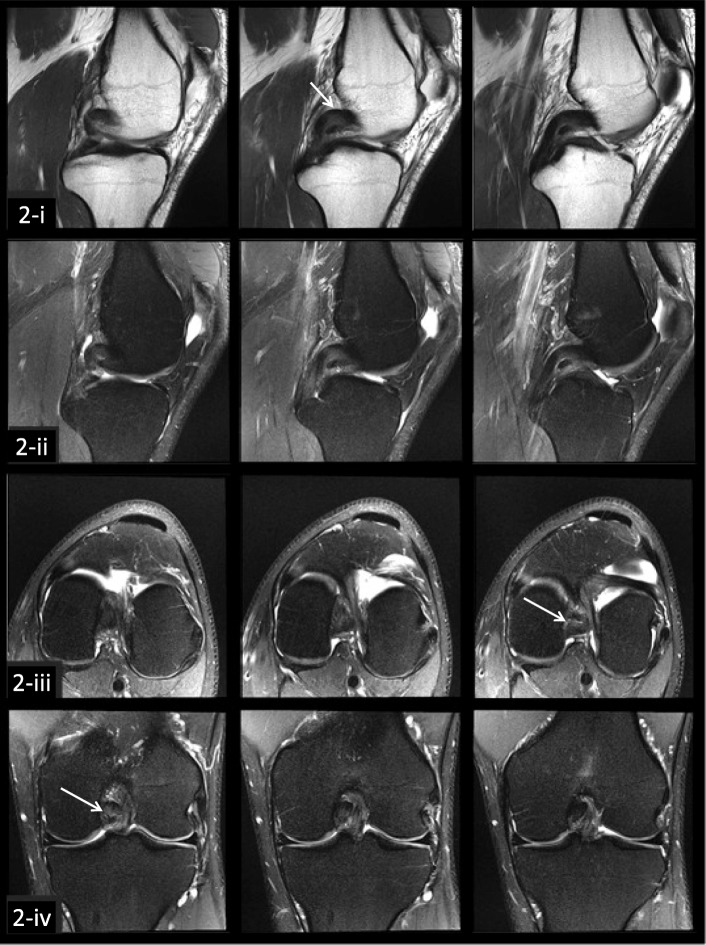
Example images of subject 2 showing three consecutive slices of the PCL from four MR imaging sequences, including i) PD TSE sagittal, ii) PD FS TSE sagittal, iii) PD FS TSE axial, iv) PD FS TSE coronal. This subject was a 28-year old male with a chronic PCL injury from a fall. On original imaging evaluation the radiologist noted minimal focal increased signal within the mid PCL substance likely indicative of mild scarring and/or degeneration. However, a secondary review of the images identified some irregularity in the proximal region of the PCL (white arrows)
